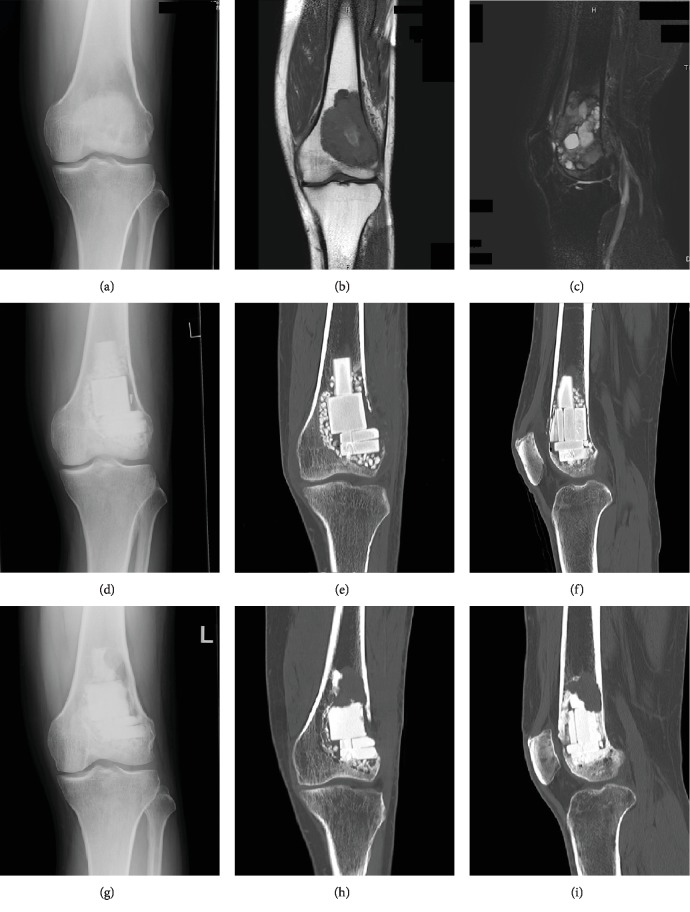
Giant cell tumor of bone in a 40-year-old male. Plain radiographs show ill-defined osteolysis in the distal femur (a). The lesion has homogenous low-signal intensity on T1-weighted images (b) and a heterogeneous intermediate- and high-signal intensity on T2-weighted images with fat suppression (c) on MRIs. The cavity after curettage is filled with *β*-TCP blocks and particles and is shown 1 month after the operation on plain radiographs (d) and CTs (e, f). Osteolysis is predominantly observed in *β*-TCP blocks on plain radiographs (g) and CT images (h, i) 1 year and 1 month after the operation.
